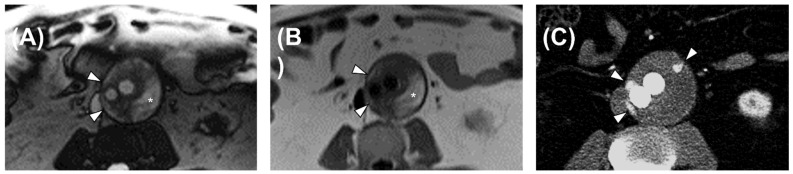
An example of a true-positive case, a 68-year-old male patient. (A) True-FISP sequence: the white arrowheads indicate hyperintensities related to the endoleak, whereas * denotes the hyperintensity of the blood clot in the aneurysmatic sac. (B) HASTE sequence: the white arrowheads indicate hyperintensities related to the endoleak, whereas * denotes the hyperintensity of the blood clot in the aneurysmatic sac. (C) CTA: arterial phase acquisition. The arrowheads indicate three hyperdense areas suggesting endoleak, one of which is not clearly visible in the RM images.
